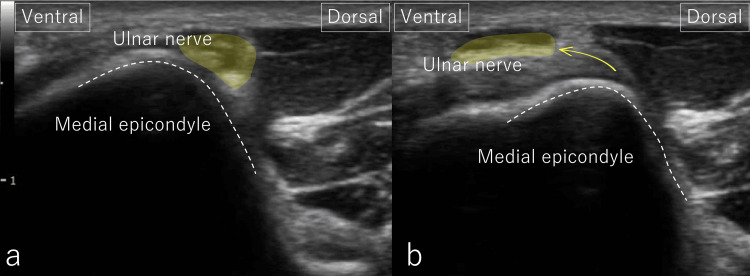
Dynamic ultrasound findings at initial presentation (short-axis view) At the cubital tunnel, the UN was dislocated over the medial epicondyle. (a) With the elbow flexed at 60°, the UN was located posterior to the medial epicondyle. (b) At 90° flexion, the UN translated anteriorly, reproducing the patient’s pain. Both images were obtained with the forearm in supination. UN: ulnar nerve
